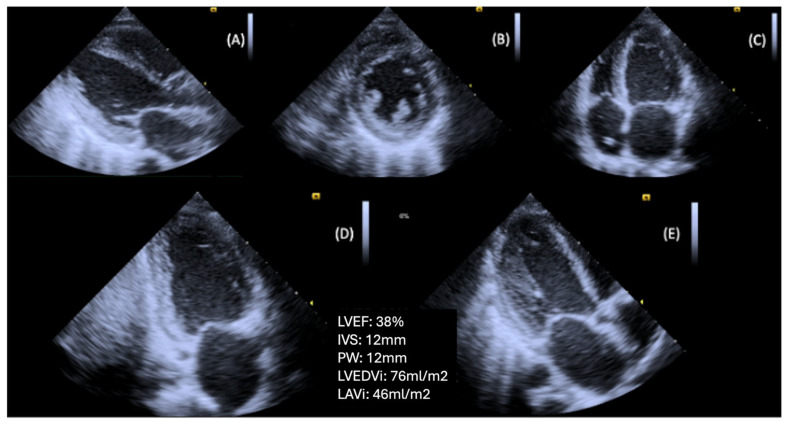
Patient with T2DM and advanced DBCM, causing left ventricular hypertrophy and systolic dysfunction. (A) Parasternal long axis view, (B) Parasternal short axis view (level of papillary muscles), (C) Apical four-chamber view, (D) Apical two-chamber view, (E) Apical long axis view (Original images from authors’ institutions).
